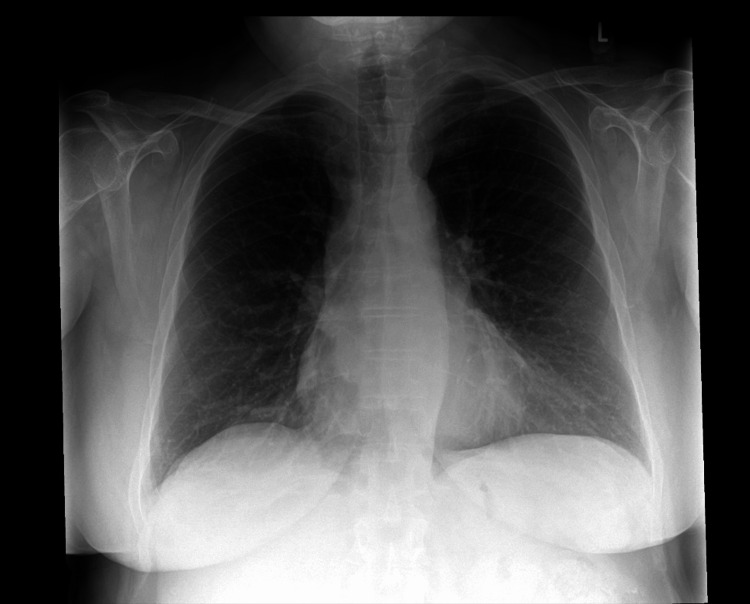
CXR demonstrating no acute lung or pleural abnormality CXR: Chest x-ray.
